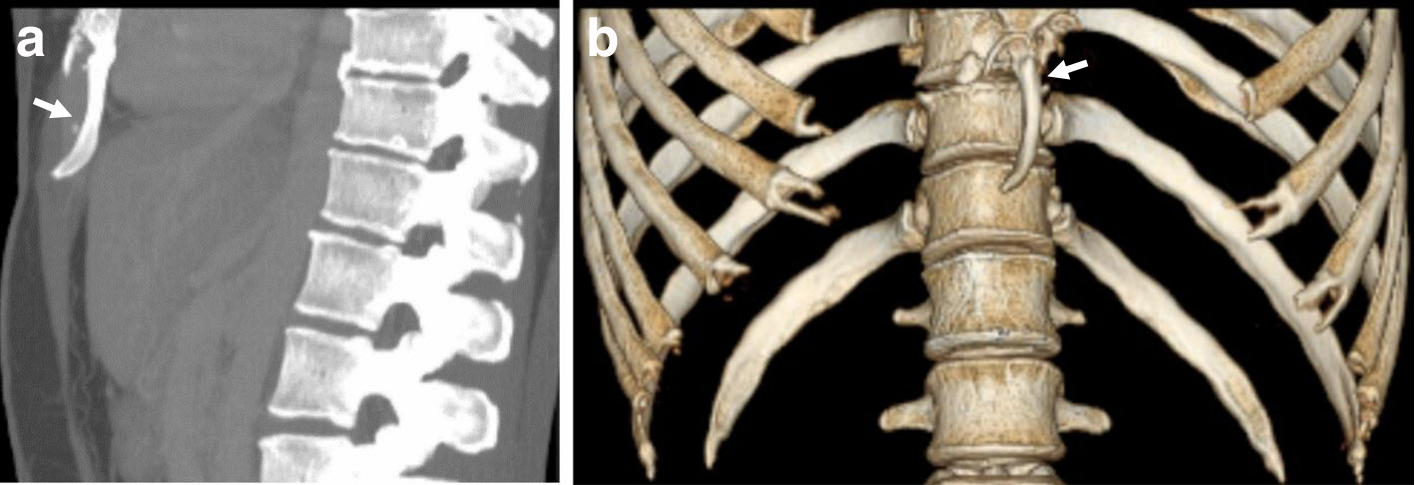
Anomaly of the xiphoid process (white arrow). a CT; b three-dimensional CT
